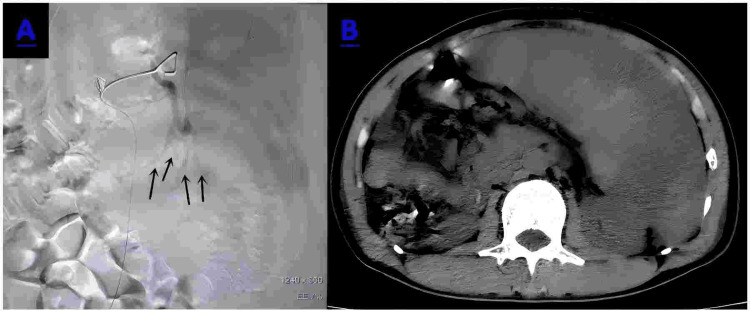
Selective splenic artery embolization procedure. A. Fluoroscopic image demonstrating occlusion of the inferior branch of the splenic artery following embolization. B. Contrast-enhanced CT image showing low attenuation of the inferior splenic pole, consistent with splenic infarction.
